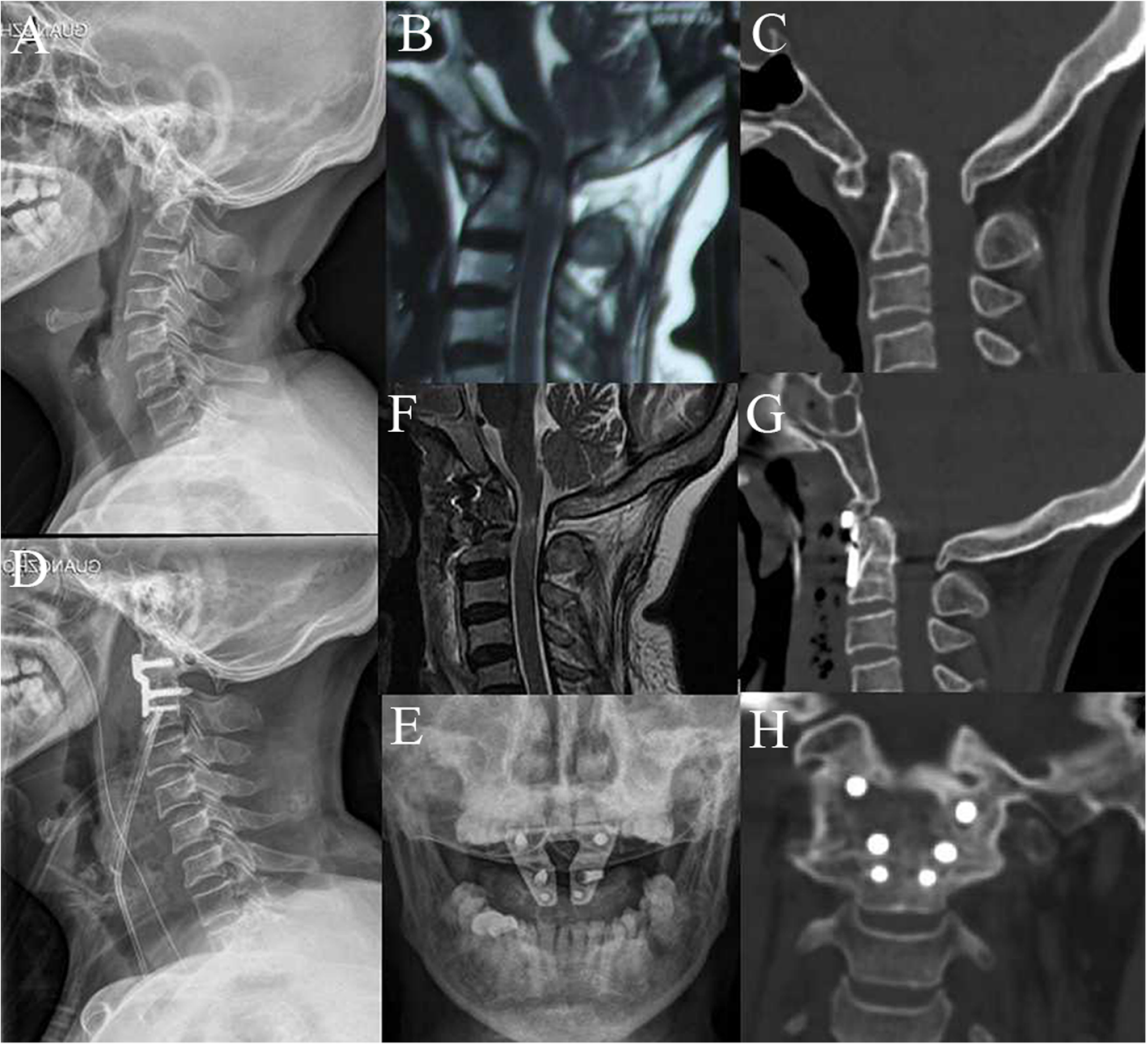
A 64-year-old woman, diagnosed with basilar invagination with atlantoaxial dislocation, underwent a TARP procedure. Images of cervical lateral radiographs (a), sagittal MRI (b), and sagittal CT scan (c) before surgery showed evidence of basilar invagination with atlantoaxial dislocation and compression on the ventral medulla. Cervical radiographs (d, e) after a TARP operation showed well placement of device. Postoperative sagittal MRI (f) and sagittal CT scan (g) showed reduction of atlantoaxial dislocation with descent of the odontoid process and decompression on the ventral medulla. CT image (h) at 3 months after surgery revealed solid bone fusion
